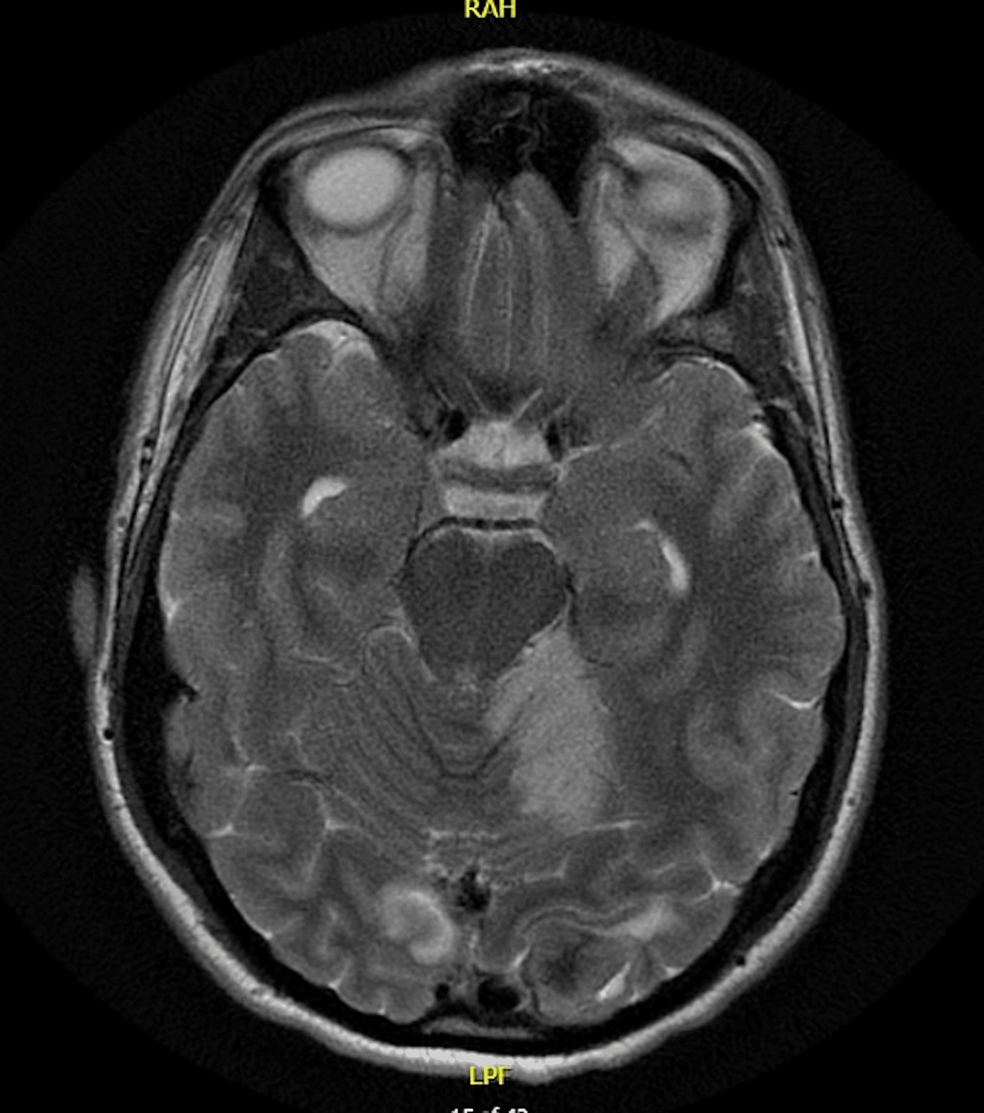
MRI of the brain showing left occipital meningioma, acute ischemia/infarction with mild hemorrhage along the left vermis and cerebellum
